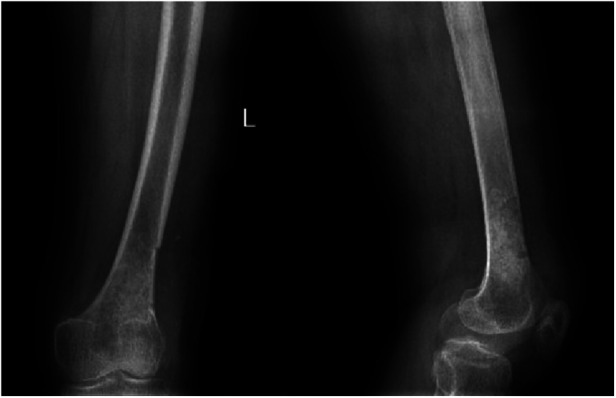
Anteroposterior and lateral radiographs of the left femur showing no abnormal signal at the left distal femur on the 3rd day after the surgery.
